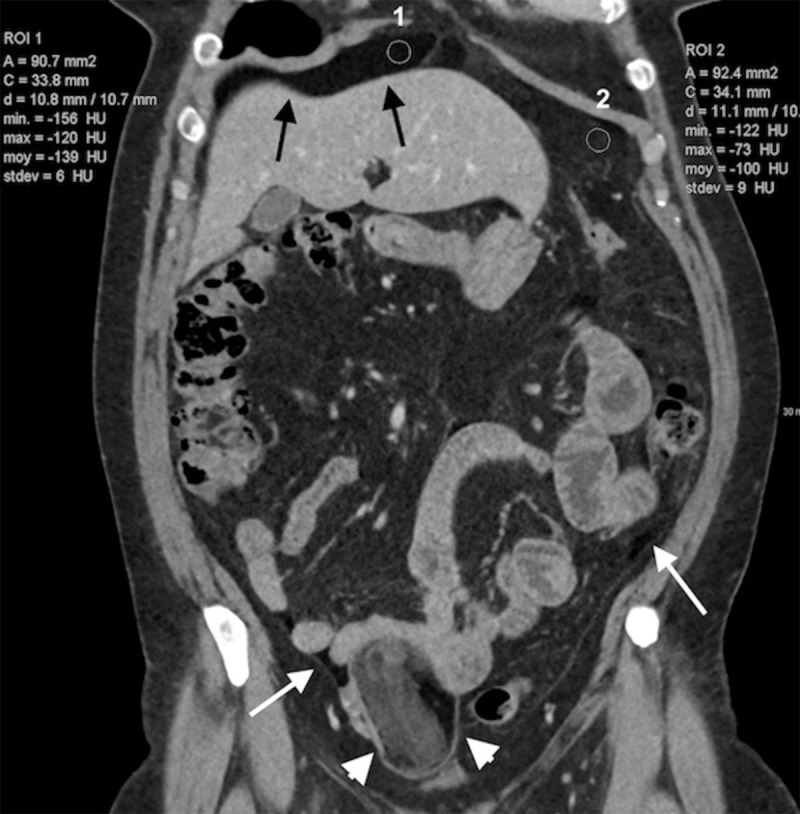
A second CT was performed three months later for persistent abdominal tenderness. It showed more organized fat zones along the mesenteric vessels, surrounding the intestine and mostly prevailing in the upper part of the abdominal cavity, under the diaphragm (Figure 2, arrows). Signs of chronic peritonitis were also visible (Figure 3), including infiltration of the intra-abdominal fat (arrows) combined with smooth thickening and enhancement of the peritoneum (arrowhead). The patient finally underwent a surgical resection confirming a ruptured right ovarian mature teratoma and diffuse oily ascites.
